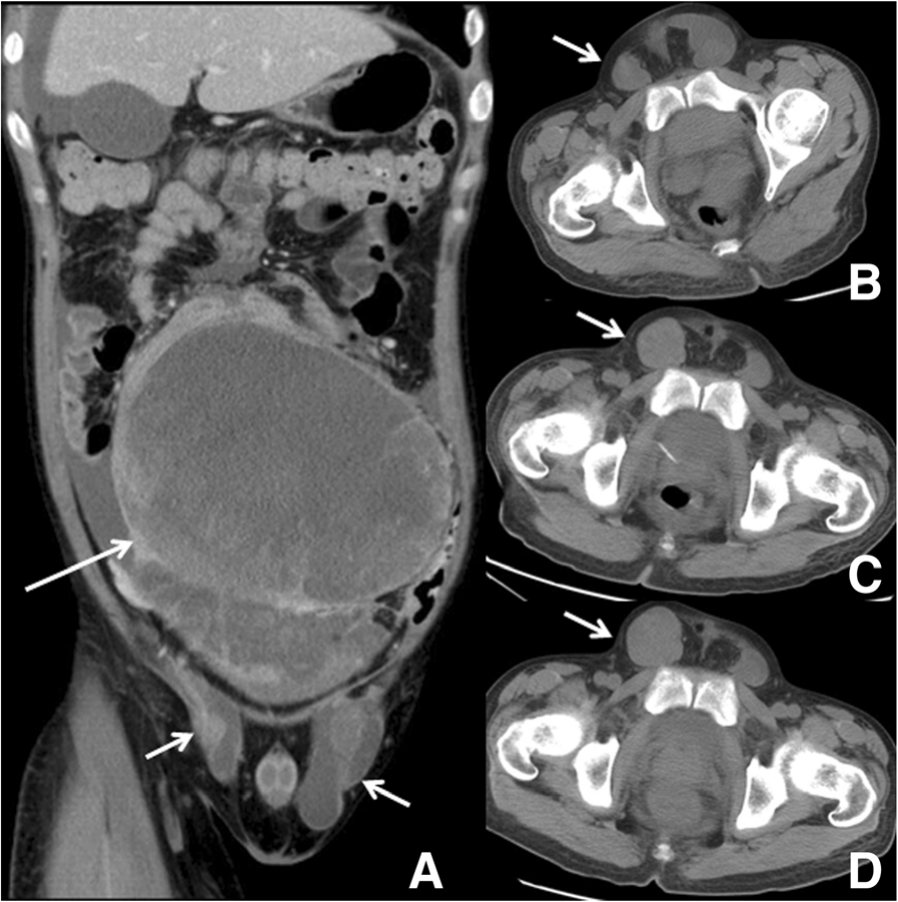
CT imaging findings of the case. a Coronal contrast-enhanced CT scan showed a retroperitoneal heterogeneous enhancement mass with multiple large necrotic areas (long arrow) and cystic-solid masses in the bilateral inguinal regions (short arrow). b-d The right inguinal region mass gradually increased (short arrow) (5 days, 18 days, 25 days after admission)
